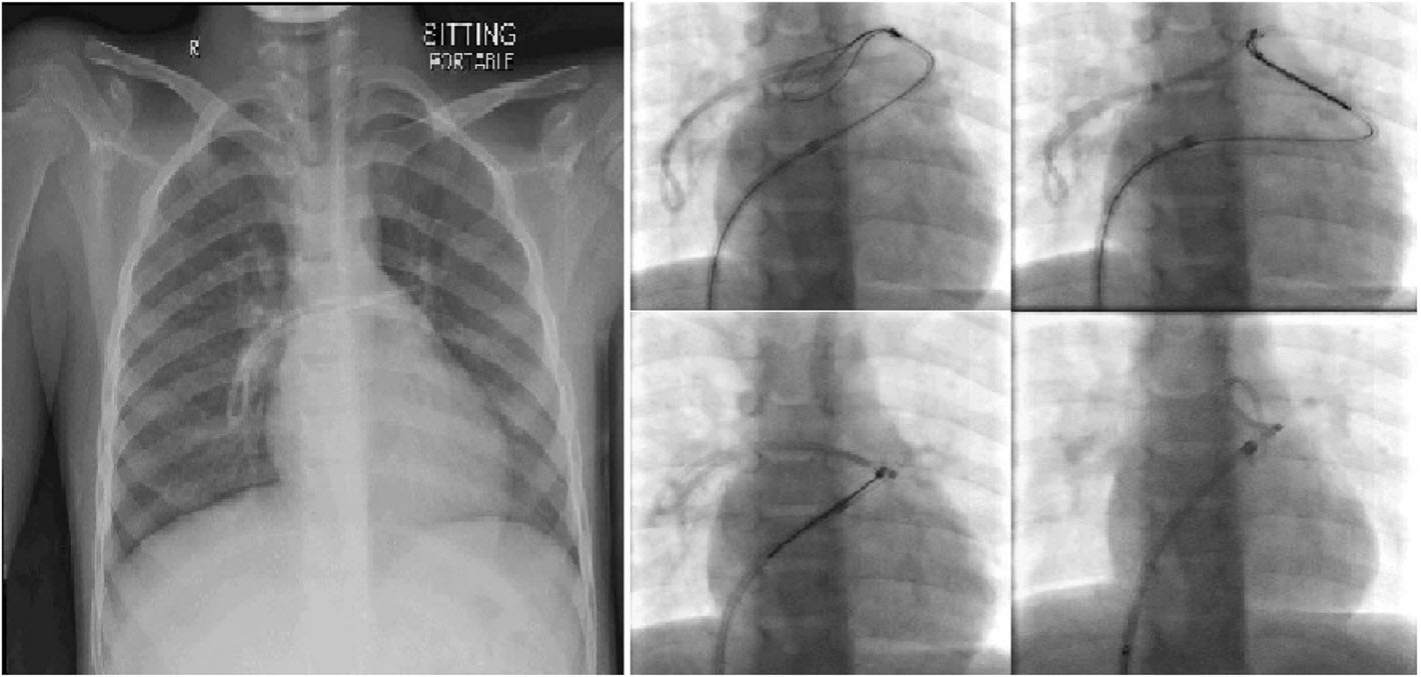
Chest X-ray and fluoroscopy showing a dislodged catheter which was retrieved by the interventional radiologists
